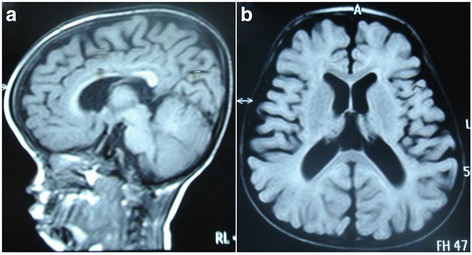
Brain MRI images showed a hypoplastic corpus callosum, enlargement of the lateral ventricles and hydrocephalus (a and b)
